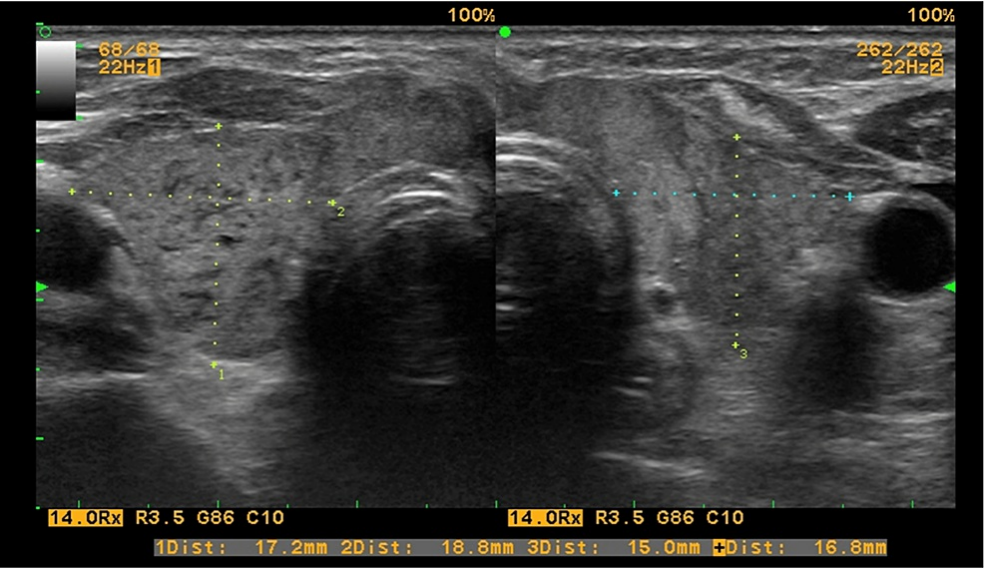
Ultrasound images of the thyroid one and a half years after the chemotherapy The thyroid gland hash slight heterogeneity of the parenchyma with small focal hypoechogenicity
